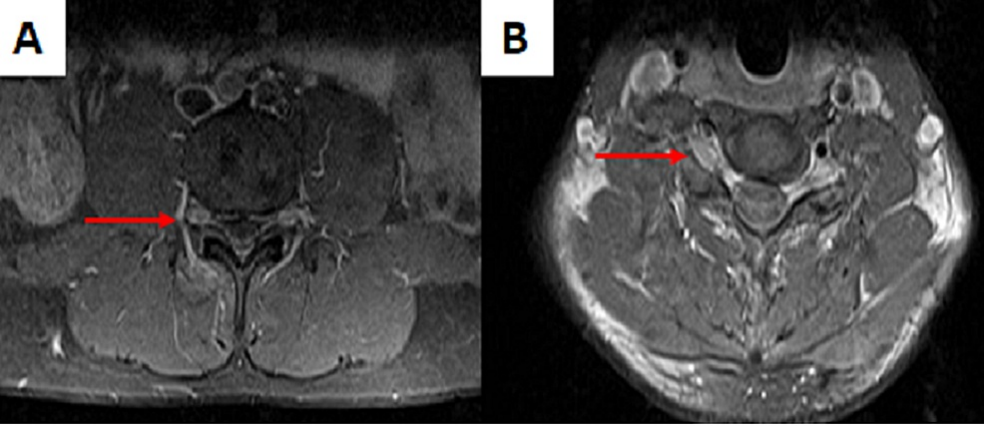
The MRI of the whole spine. Enlarged dorsal root ganglia (arrows) at the lumbar (A) and the cervical (B) regions (T1W gadolinium-enhanced, transverse view).
